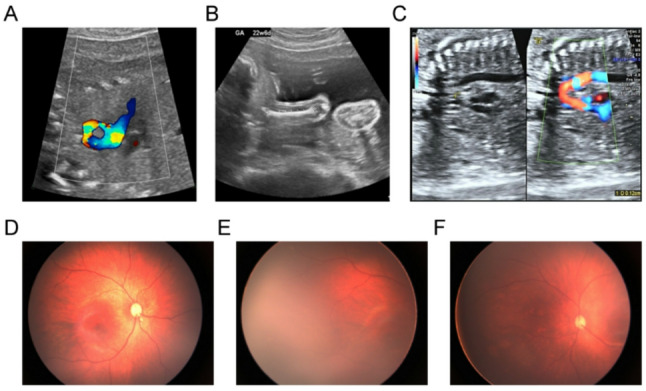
Clinical manifestations of the affected individuals. A Prenatal ultrasound at 23 weeks of gestation in P1 revealed pulmonary atresia with ventricular septal defect and overriding aorta. B At 22^+ 6^ weeks of gestation, ultrasound evaluation of P2 demonstrated femoral shortening, indicating impaired femoral development. C Prenatal ultrasound at 22 weeks of gestation showed P4 had aortic stenosis and ventricular septal defect. Ophthalmologic examination of Patient 5 revealed a choroidal coloboma (D) and punctate white lesions in the temporal retina of the right eye (E), and pigmentary alterations consistent with retinal dystrophy in the left eye (F)
